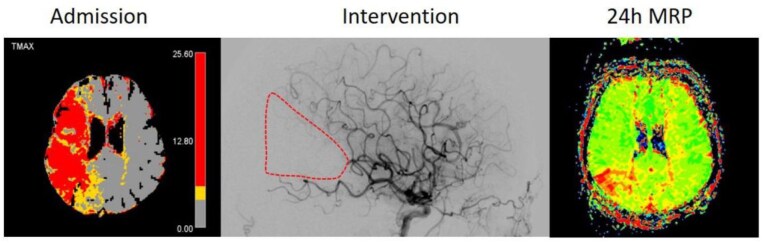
Late reperfusion assessment. Evaluation of 24 h MRI perfusion using Time to Peak (TTP) maps. Patient with initial M1 occlusion an eTICI2b67 reperfusion after MT. Follow-up MRI at 24 h reveals a wedge-shaped TTP deficit, meaning that complete late reperfusion has not occurred. For this patient the co-primary endpoint of late reperfusion was rated as negative/no late reperfusion.
